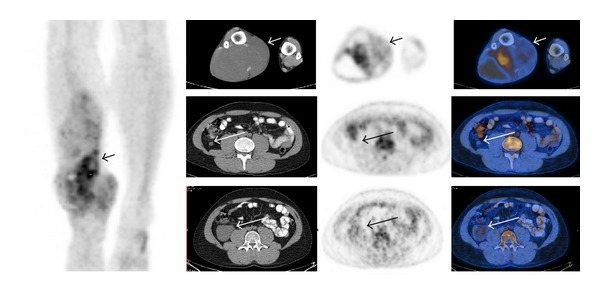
True positive in this 58-year-old patient with malignant peripheral nerve sheath tumor of the right thigh (long arrow). The FDG-PET scan demonstrated two additional hypermetabolic lesions in the left calf (short arrow). These were subsequently resected and proven to be synchronous peripheral nerve sheath tumors. Four years later, the patient is in remission and is doing well.
